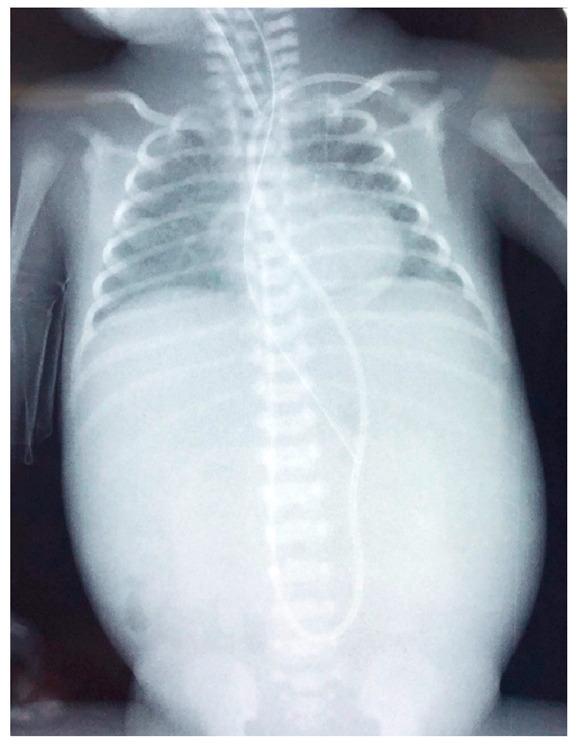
Radiography of the infant on the day of deterioration, with non-specific signal characteristics in abdomen, such as distended abdomen and poor distribution of bowel gas.
